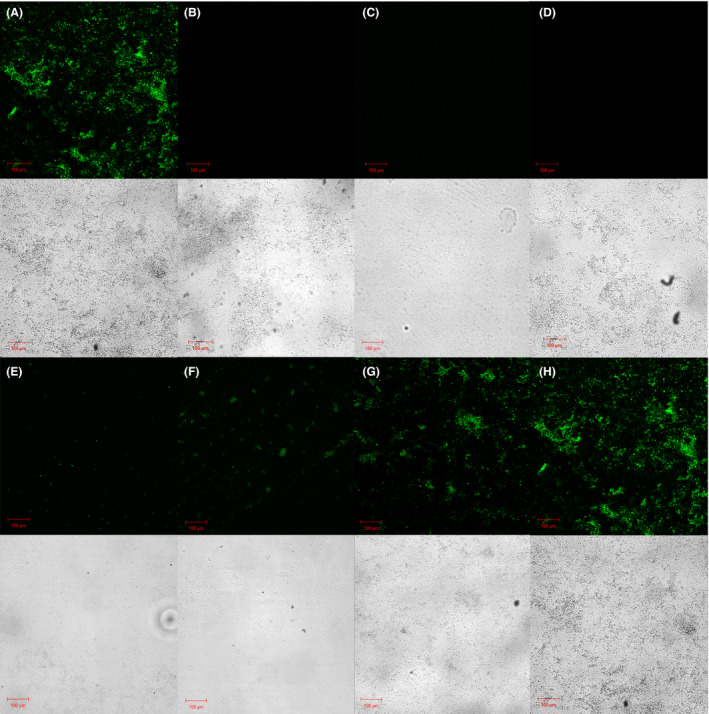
Time course of ultrasound‐mediated gene transfer into a biofilm. Green fluorescence signal (ex. 485nm, em. 510nm) and bright‐field imaging of biofilm samples after 120 h with (A) the addition of both plasmid and ultrasound treatment (+P/+U), (B) only the addition of plasmid (+P/−U), (C) only ultrasound treatment (−P/+U) and (D) no plasmid and no ultrasound treatment (−P/−U). Green fluorescence signal and bright‐field imaging for biofilm samples with both addition of plasmid and ultrasound treatment after (E) 5 h, (F) 24 h, (G) 48 h and (H) 120 h.
